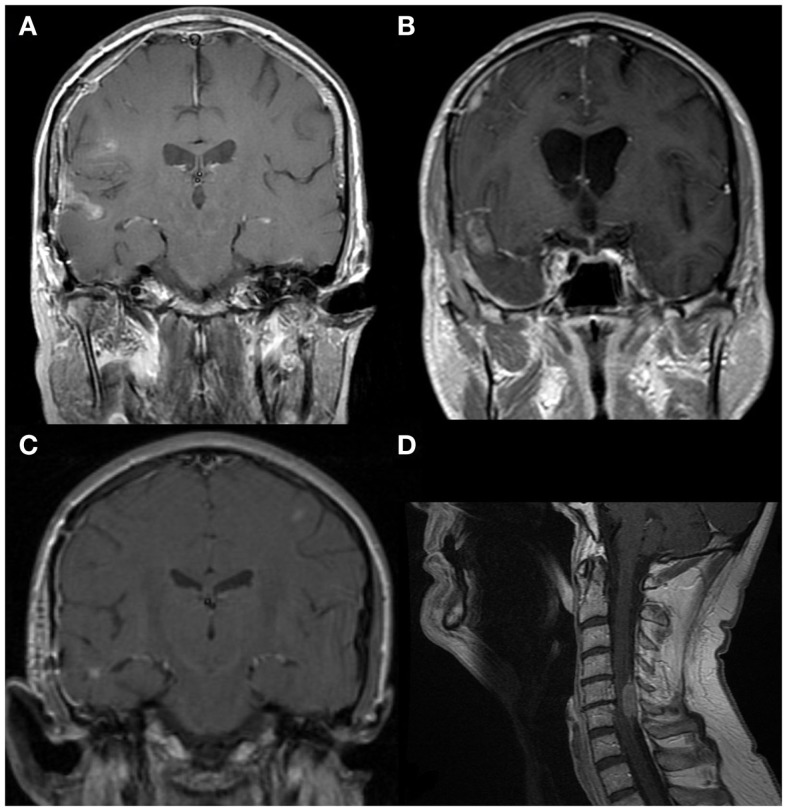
MRIs (1.5T, T1-weighted, post-contrast). (A) GBM with local spread to the right superior temporal sulcus. Further spread rostrally along the juxta-cortical leptomeningeal space is present. (B) GBM with local spread to the right transverse sulcus. Further spread rostrally along the juxta-cortical leptomeningeal space can also be seen. (C) A small focus of GBM in the right temporal lobe with spread to the right middle temporal sulcus, then rostrally along the subarachnoid space on the right. A second focus is seen in the left middle frontal gyrus. This is likely to have spread through the leptomeningeal space. (D) GBM with spread to dura at C5–6 and minimal intramedually invasion. Intrathecal chemotherapy is unlikely to penetrate this fully and flow caudally is likely to be impaired.
